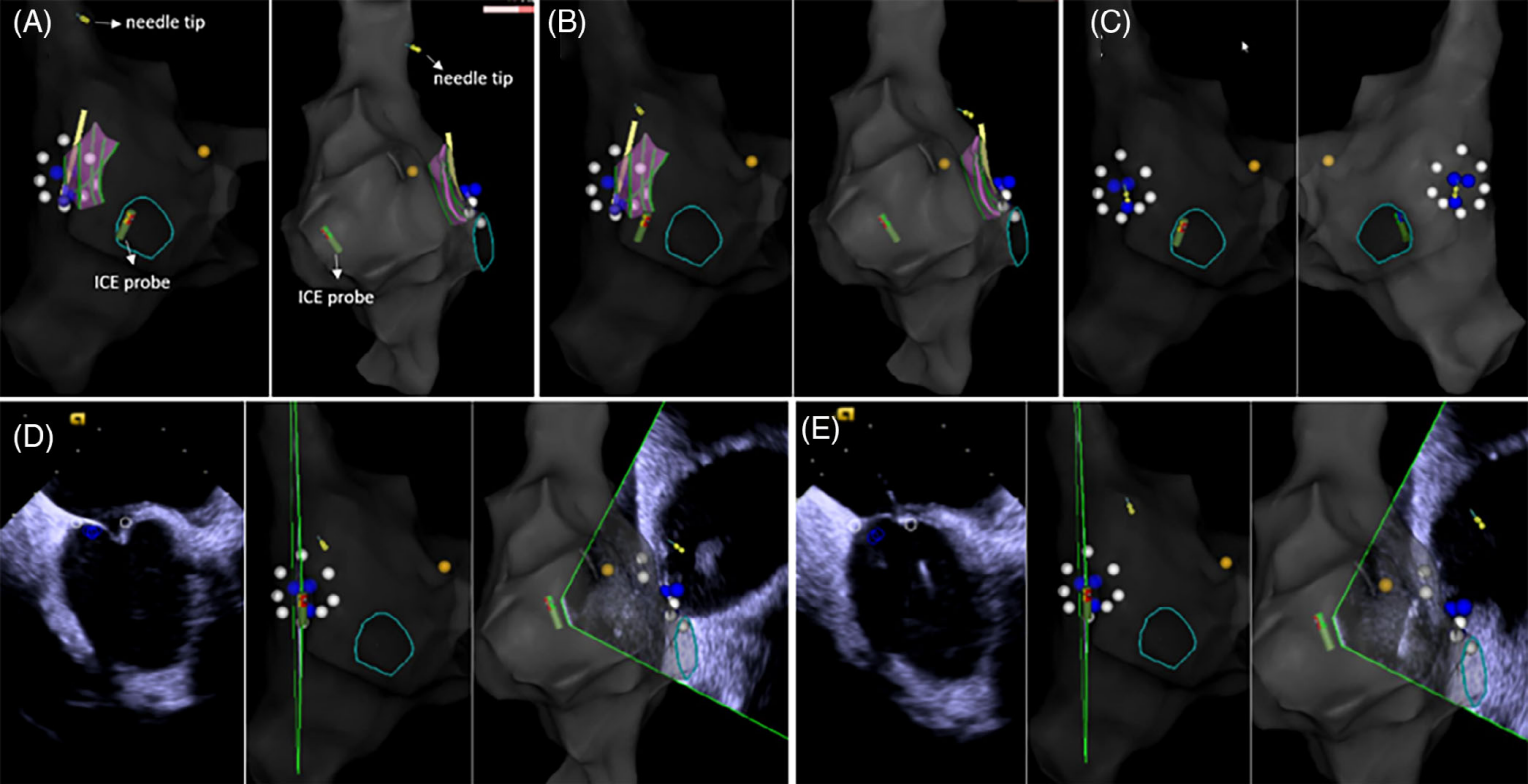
Transseptal puncture with electroanatomical mapping combined with diagnostic ultrasound catheter (EAM‐ICE). A, The long sheath was placed into the SVC through the guide‐wire, which was then replaced with the trans‐septal needle. The tip of the needle was inserted into the SVC (yellow circle). B and C, After withdrawal of the sheath‐dilator‐needle assembly, the tip the needle was oriented toward the atrial septum. The needle can be seen facing toward the septum in C. D, Under ICE direction, the trans‐septal needle was used to puncture the atrial septum. When the trans‐septal needle was pushed toward the atrial septum, a tenting phenomenon could be seen on ICE. E, After a rapid movement of the transseptal needle through the dilator succeeded in puncturing the atrial septum, a small bolus of physiological solution was used to confirm that the needle was inside the left atrium. The shadow created by the bolus can be seen on ICE. (yellow circle, tip of the trans‐septal needle; yellow point, bundle of His; white point, foramen ovale; blue point, low‐voltage area)
